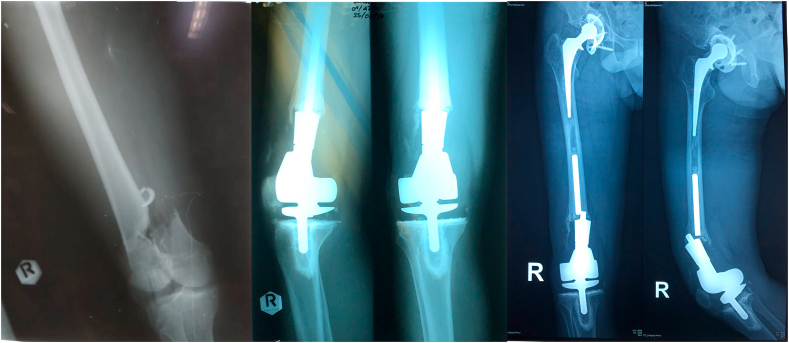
Serial X-ray of the right knee.
